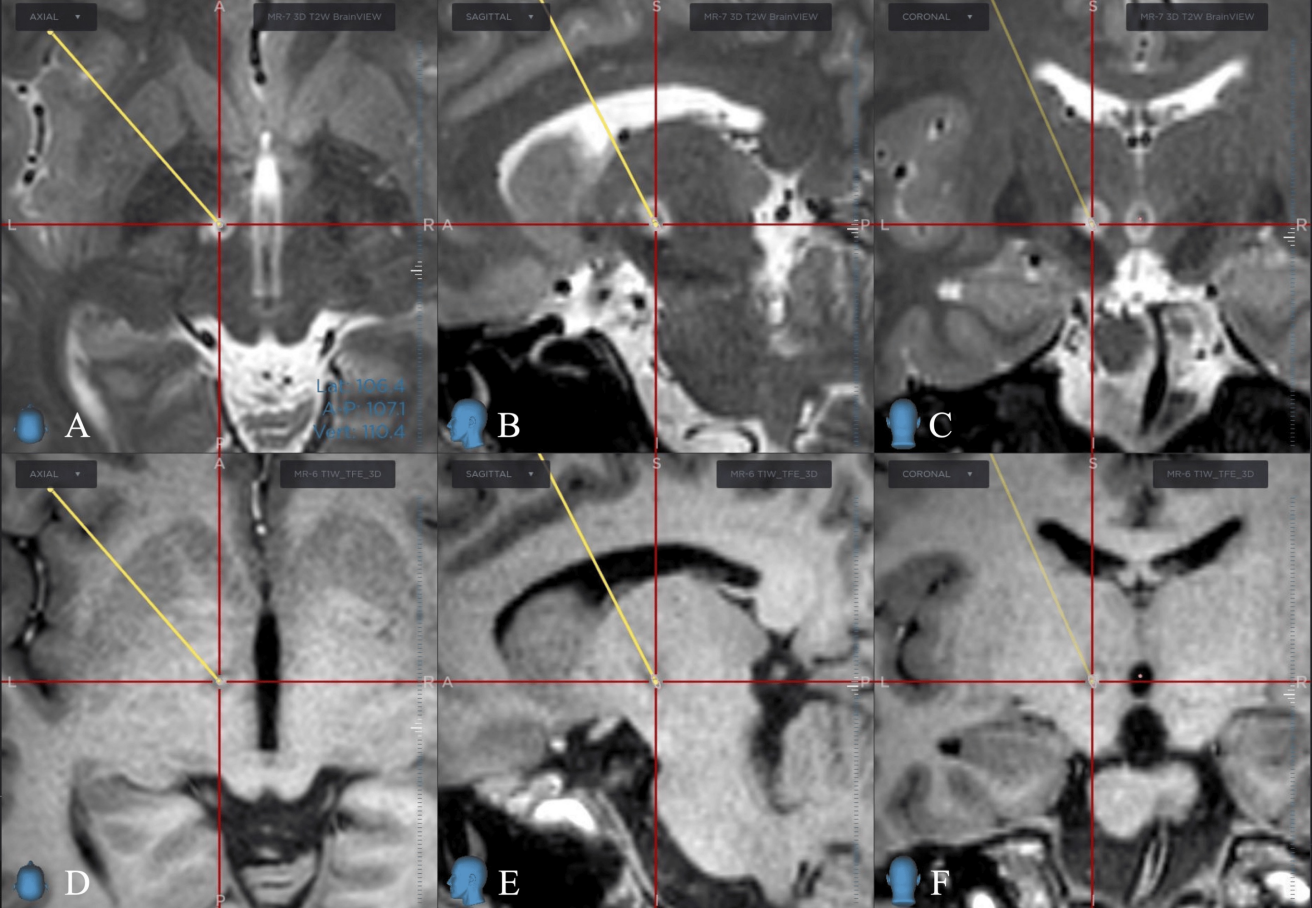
Postoperative MRI. The planned target and the coagulation point are almost in the same location. (A) Axial, (B) sagittal, and (C) coronal sections on T2-weighted imaging (T2WI). (D) Axial, (E) sagittal, and (F) coronal sections on T1-weighted imaging (T1WI). The yellow line shows the planned trajectory, and the intersection of the red lines, i.e., the center of each panel, is the target set preoperatively.
